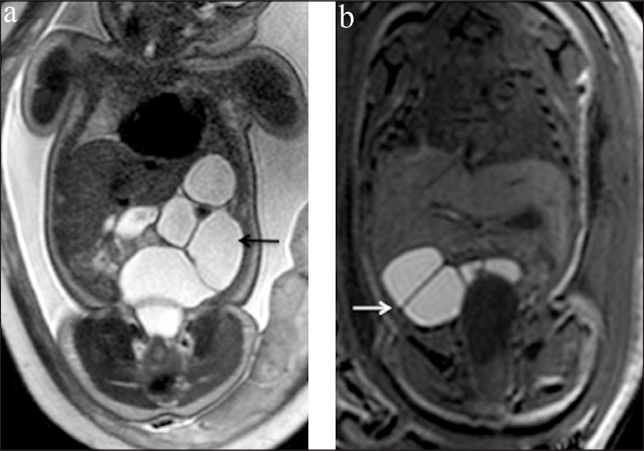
Differentiation between proximal and distal small bowel occlusion by bowel signal content. (a) Coronal T2-weighted sequence on the abdomen of a 32 week-old fetus with distended hyperintense loops (arrow) in the left hypochondrium corresponding to dilated proximal small bowel filled with ingested amniotic fluid in a context of proximal small bowel atresia. (b) Coronal T1-weighted sequence on the abdomen of a 37 week-old fetus with distended hyperintense loops (arrow) in the right flank corresponding to dilated distal small bowel filled with meconium in a context of ileal atresia.
